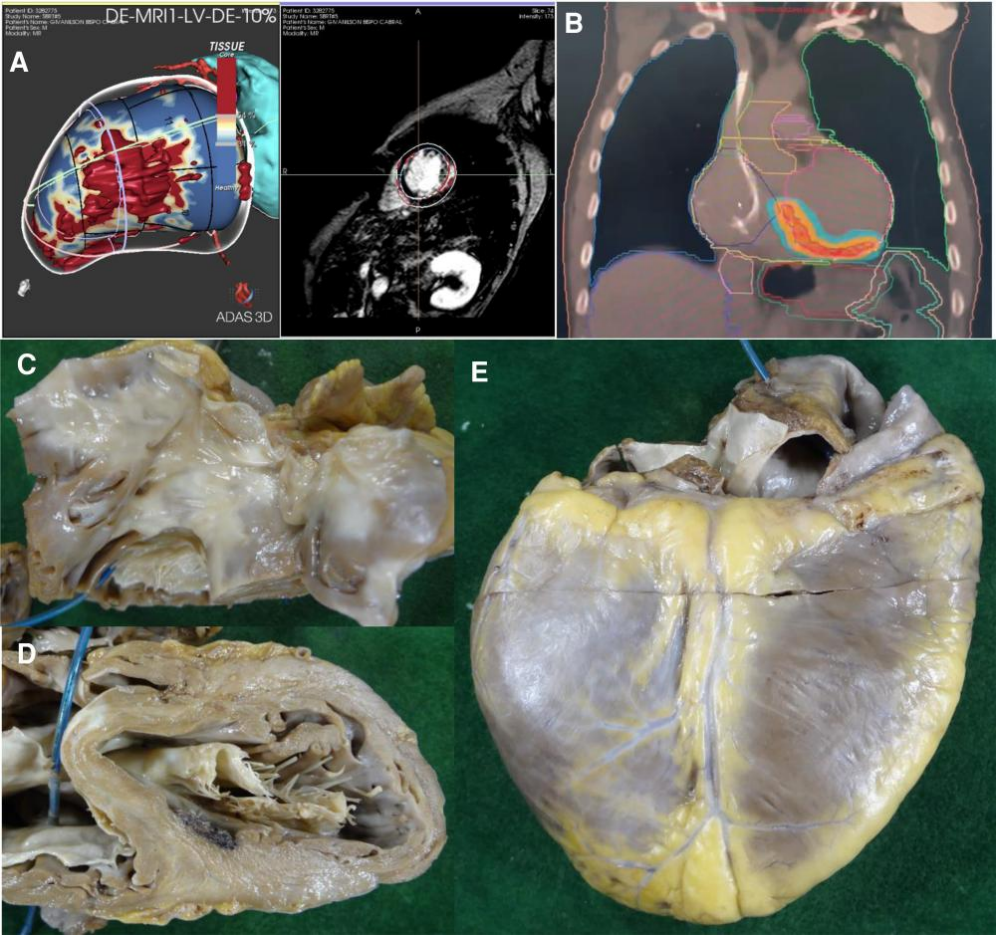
(*A*) 3D reconstruction of coronary computed tomography angiography (ADAS 3D®, Adas3D Medical SL, Barcelona, Spain) showing a scar in the mid-lateral region of the left ventricle. Induced ventricular tachycardias suggested septal and inferior exits; therefore, the irradiated area (*B*) included the entire inferior left ventricle region. (*C*) Macroscopic view of radiofrequency lesions in the right ventricular outflow tract related to previous ablations. (*D*) Area of necrosis in the basal septal left ventricle region—this region underwent both radiofrequency (RF) ablation and stereotactic arrhythmia radiotherapy. (*E*) Whitish area in the mid-basal inferior left ventricle wall, corresponding to the irradiated area (blue arrows). VT, ventricular tachycardia.
